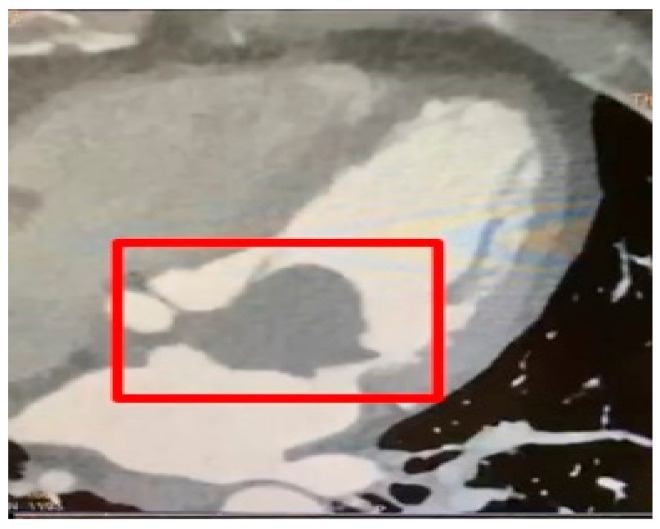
The echocardiogram test revealed a large left atrial mass (red square) measuring 3.6 × 4.2 cm which was attached to the interatrial septum.
